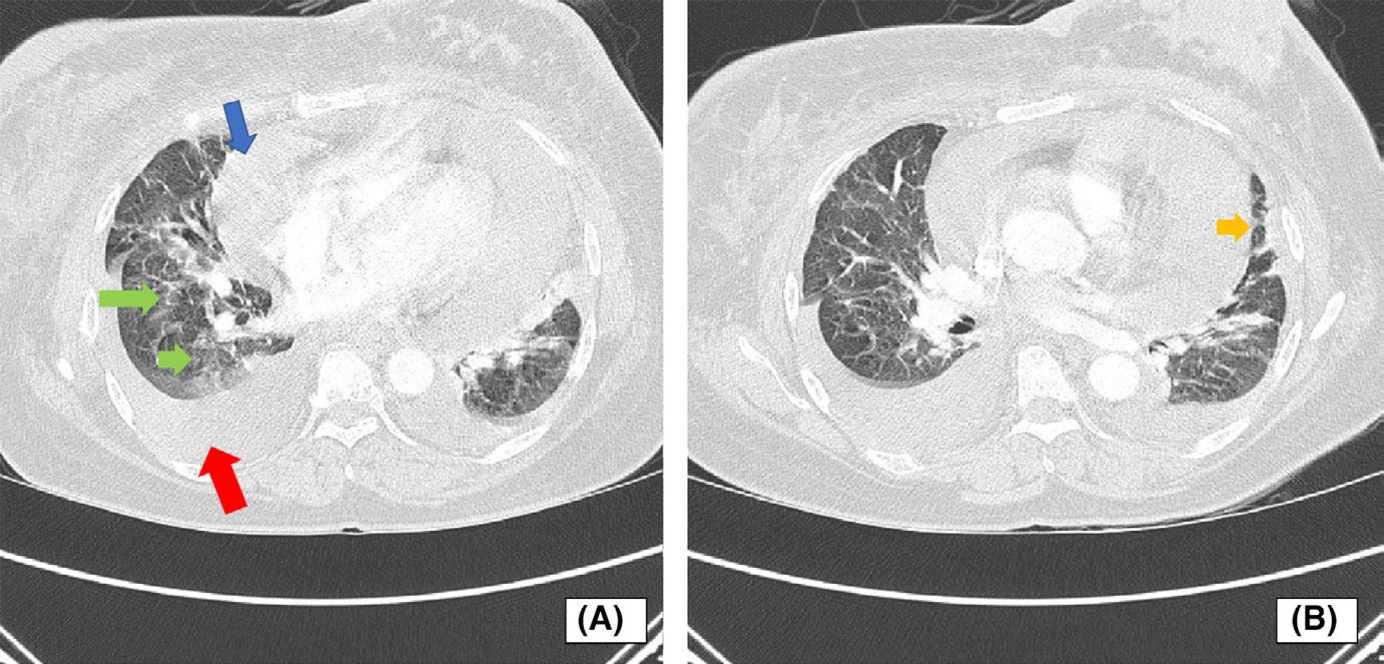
A and B, Thoracic CT scan showed pleural effusion (red arrow), pericardial effusion (blue arrow) and pneumonic type pulmonal metastases (green arrow) and subpleural type pulmonal metastases (yellow arrow). It also showed a left breast mass with irregular shape that extended to the chest wall and infiltrated to the skin and major pectoralis muscle
